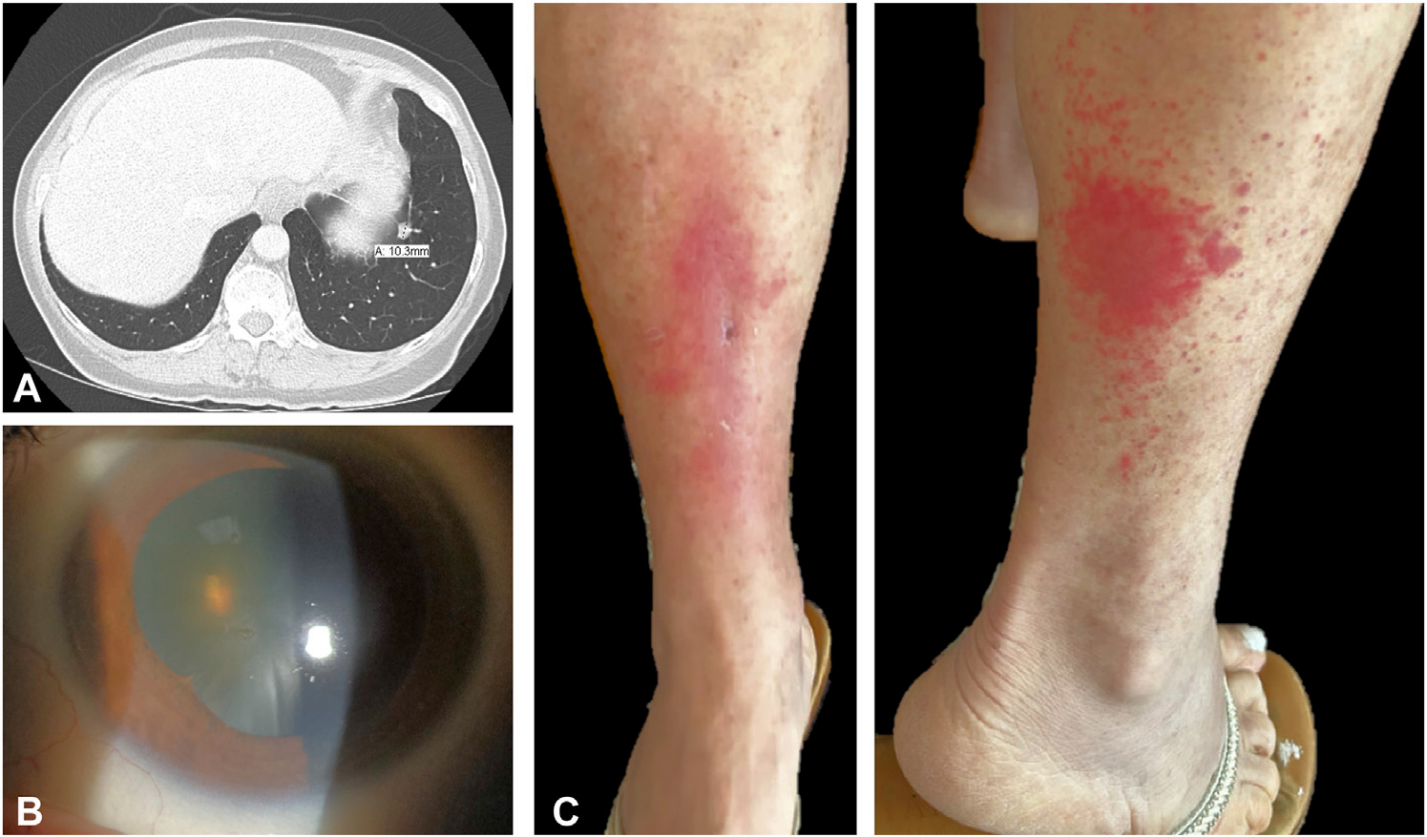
A, Computed tomography of the chest showing a 1 cm pulmonary micronodule. B, Sequela of anterior uveitis in the form of a focal posterior synechiae in the left eye. C, Poorly defined, erythematous non-scaly patch on the anterior leg, and brightly erythematous papules centrally coalescing into a plaque clustered on the posterolateral leg.
